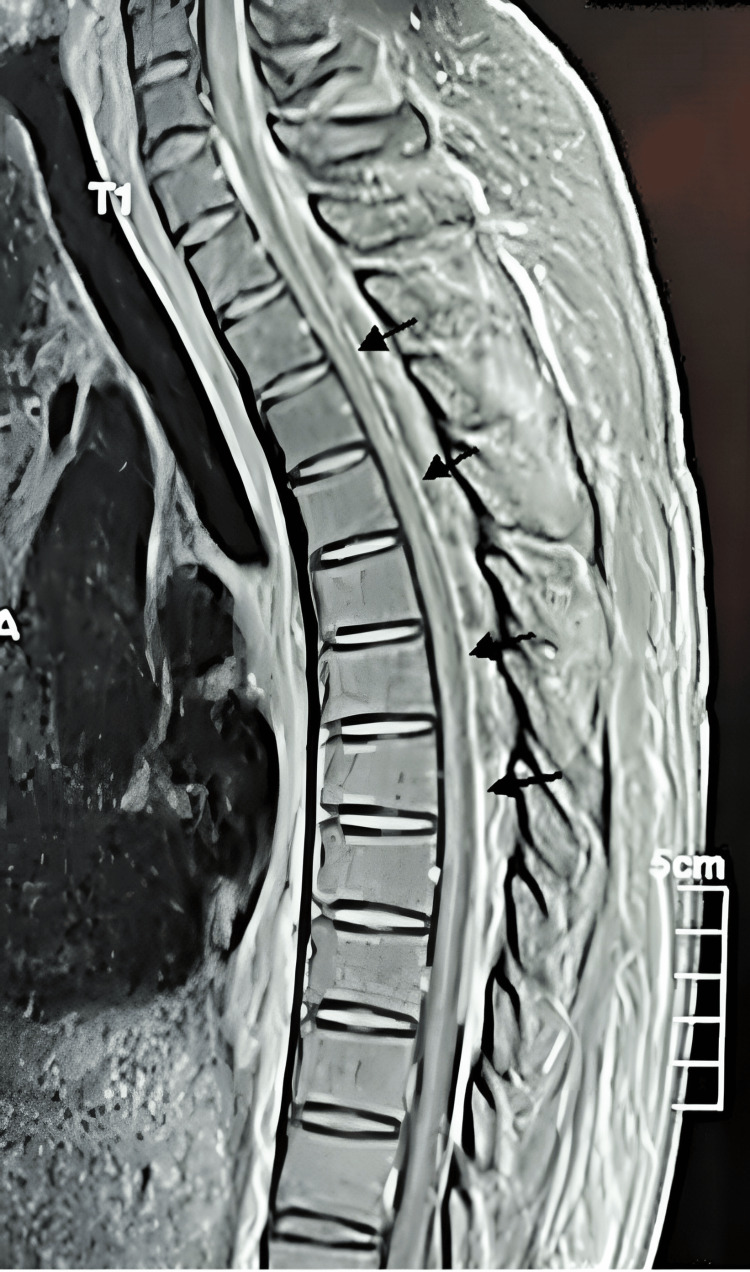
Sagittal T1-weighted MRI with gadolinium administration The image shows a heterogenous cervicothoracic spinal epidural abscess at C6-T10 with hypointense and isointense images in the posterior region of the spinal canal and hyperintense linear enhancement, predominantly in the ventral region (arrows) MRI: magnetic resonance imaging
